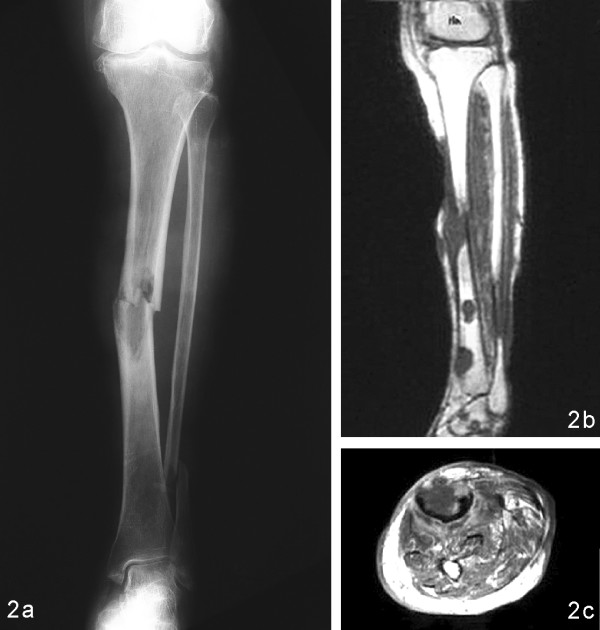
(a). Plain radiograph of a 74-year-old woman. The radiograph reveals a pathologic fracture at the diaphysis of the left tibia due to an osteolytic lesion in the medullary cavity. (b). T1-weighted magnetic resonance image showing several tibial lesions that are eccentric, oval and associated with clinically significant cortical erosion. (c). T1-weighted magnetic resonance image demonstrating a hypointense lesion with endosteal scalloping of the tibia and cortical erosion.
